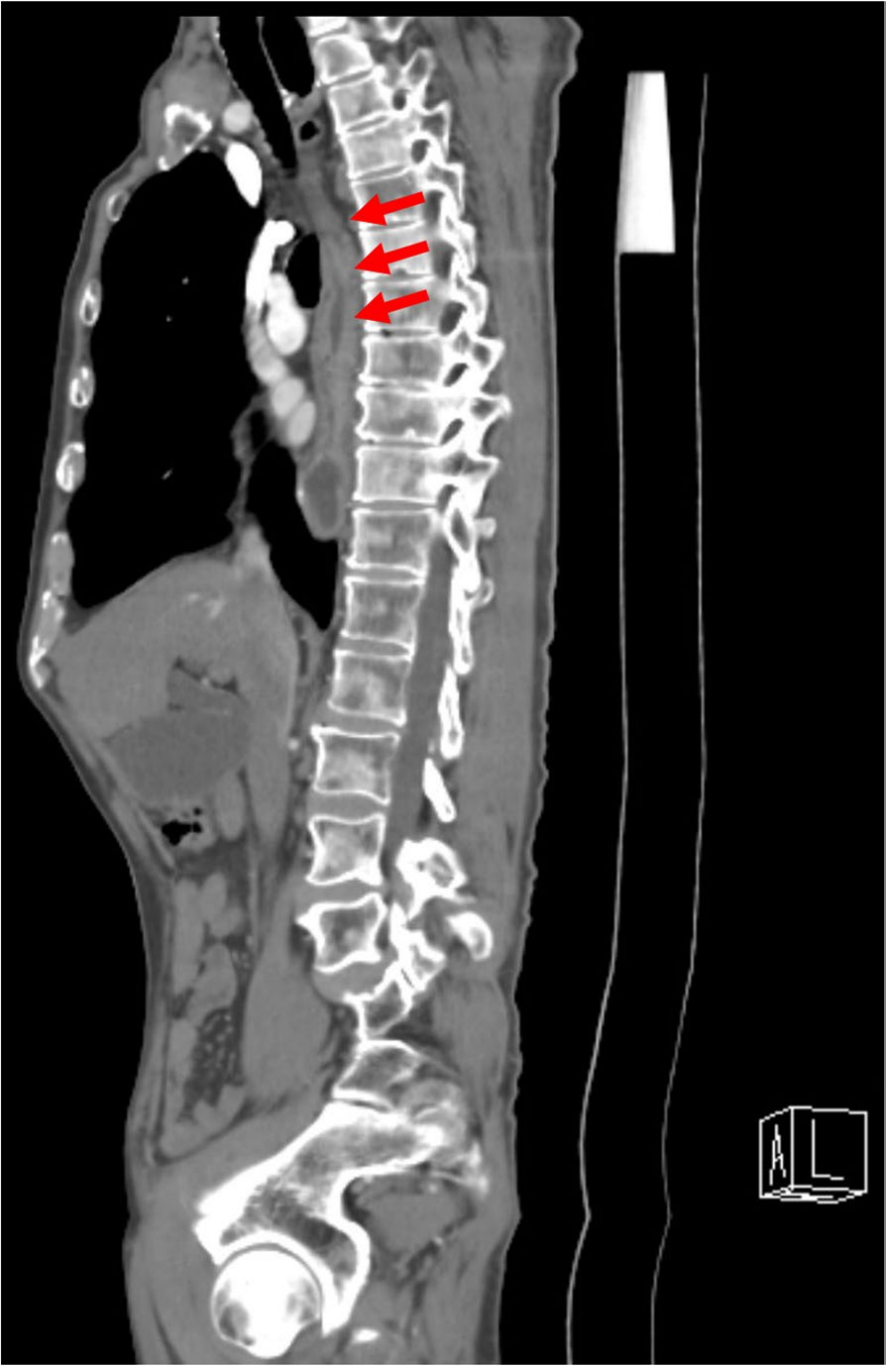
Preoperative computed tomography. The esophagus was reconstructed in the posterior mediastinal tract (red arrows), and there was no finding of recurrence
